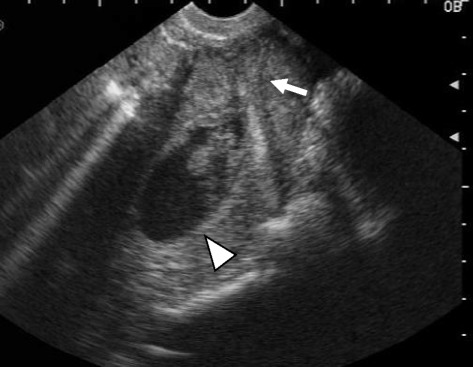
Preoperative ultrasound of patient 1. Hematometra is shown. *Arrow* and *arrowhead* represent cervix and hematometra, respectively
